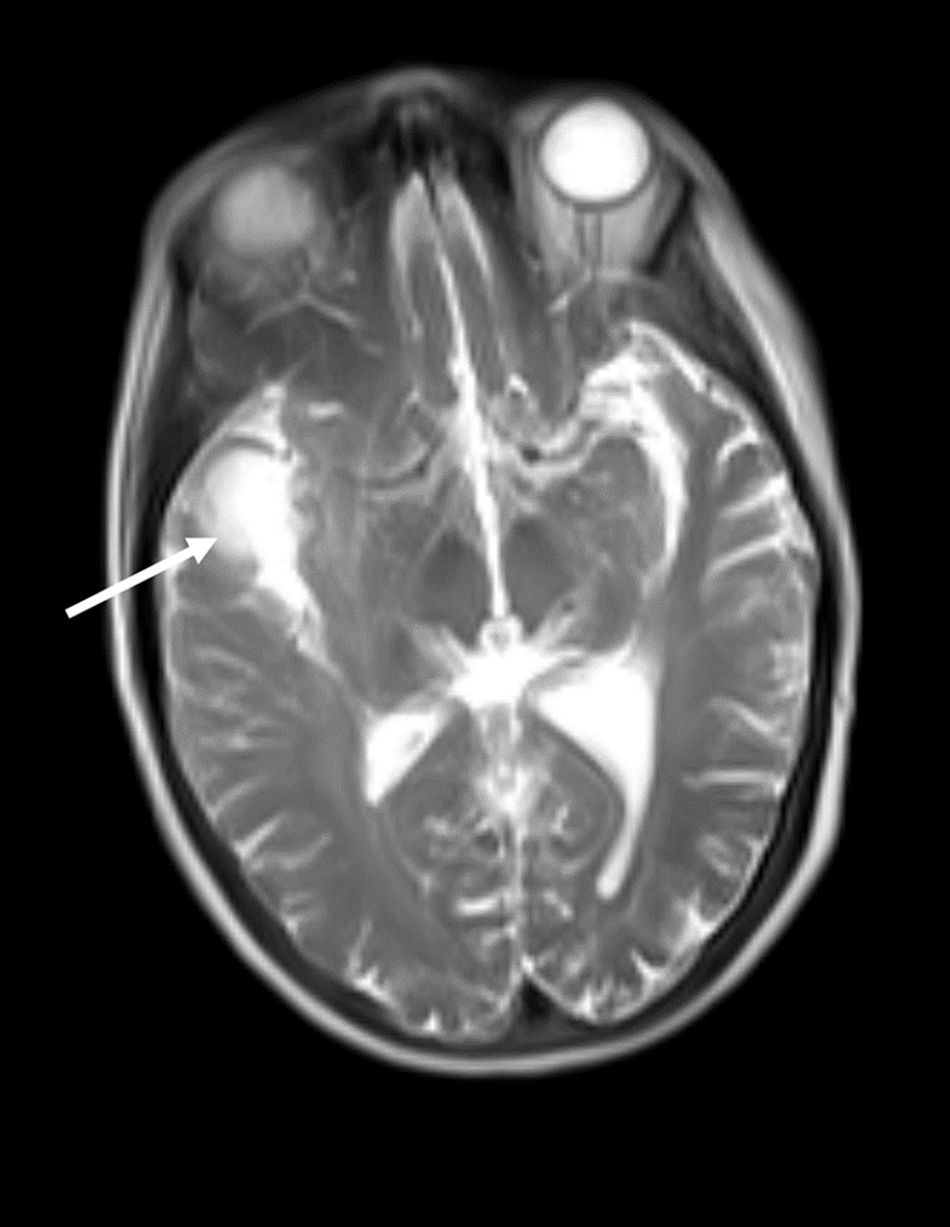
MRI image showing an expansive lesion in the right frontal region measuring 58 × 38 × 50 mm.
